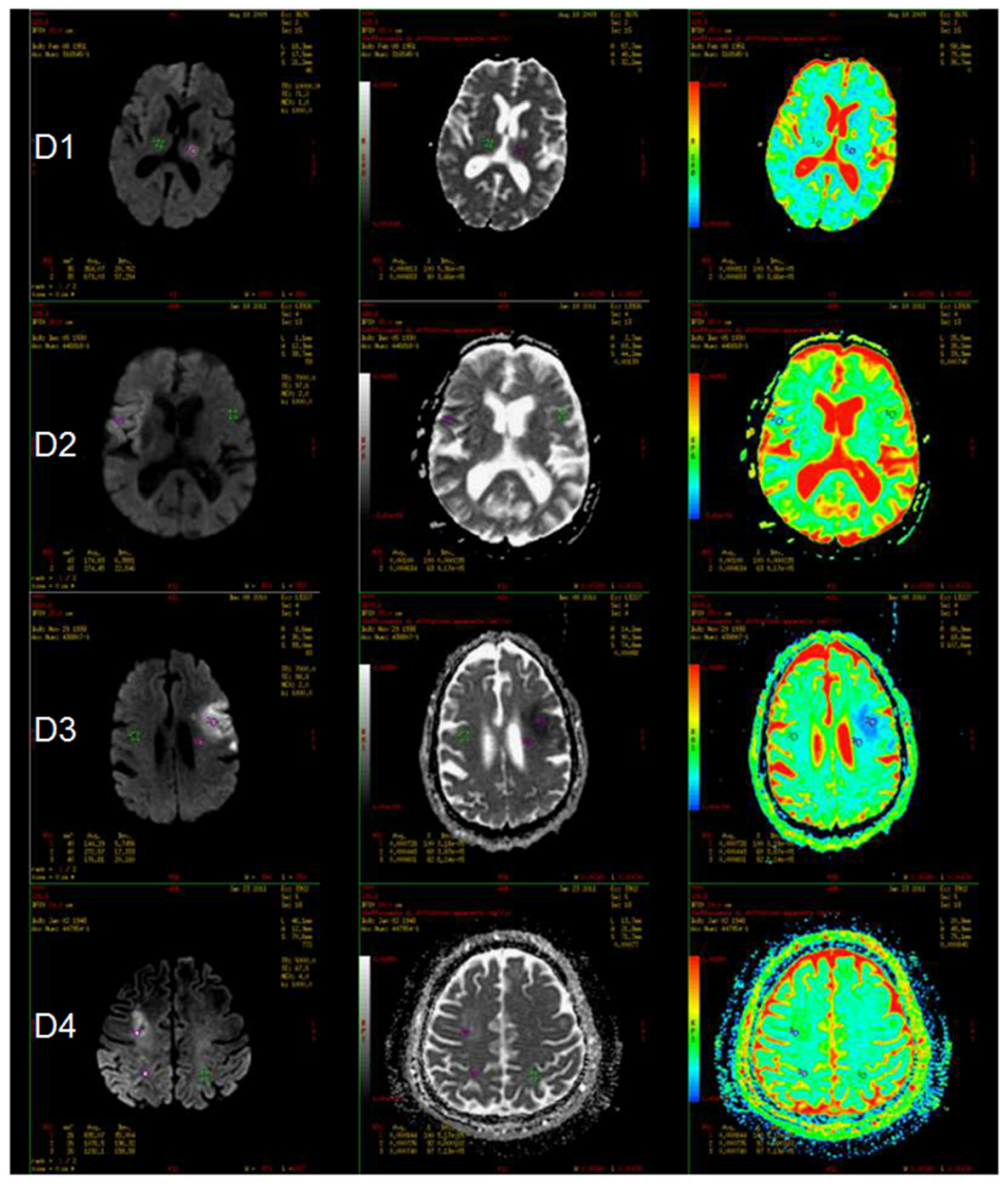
Axial diffusion-weighted imaging (DWI) and apparent diffusion coefficient (ADC) maps in grey level and multi-chromatic that show acute ischemic lesion (D1 - D4): a lesion that is hyperintense on DWI and hypointense on ADC map is a characteristic magnetic resonance imaging (MRI) finding in acute ischemic infarction. Note: regions of interest (ROI) positioned on normal parenchyma (1, green) and on acute ischemic areas (2,3, purple/red) in order to demonstrate hyperintensity on DWI corresponding to hypointensity on the ADC map in grey level. Multi-chromatic ADC maps show blue/light green areas in correspondence of ischemic lesions.
